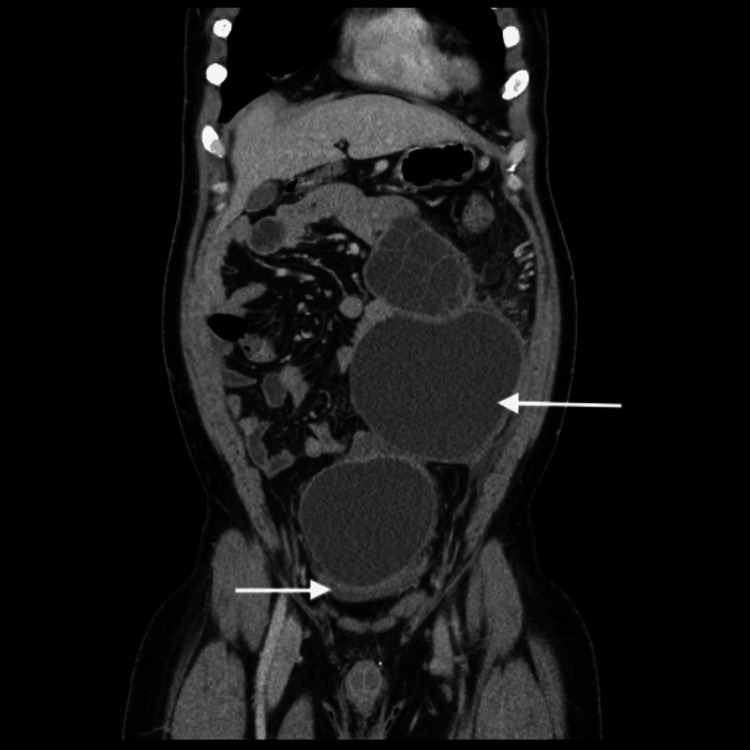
Abdominopelvic CT scan image (coronal view) Coronal CT scan image shows multiple intra-abdominal and pelvic cystic lesions consistent with secondary peritoneal dissemination. A large pelvic cyst is seen compressing the bladder (lower arrow).
